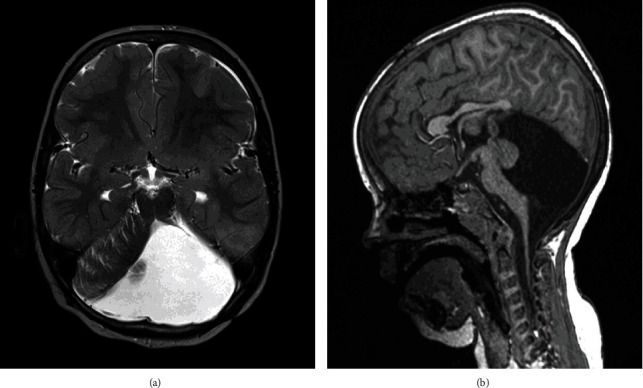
Axial T2 (a) and sagittal T1 (b) MRI scans show hypoplasia of the cerebellar vermis with cephalad rotation of the vermian remnant, cystic dilatation of the 4th ventricle extending posteriorly, anterior displacement of the cerebellar hemispheres, and enlargement of the posterior fossa. The torcula lies higher than the level of the lambdoid suture due to the abnormally high tentorium which is opposite of normal.
